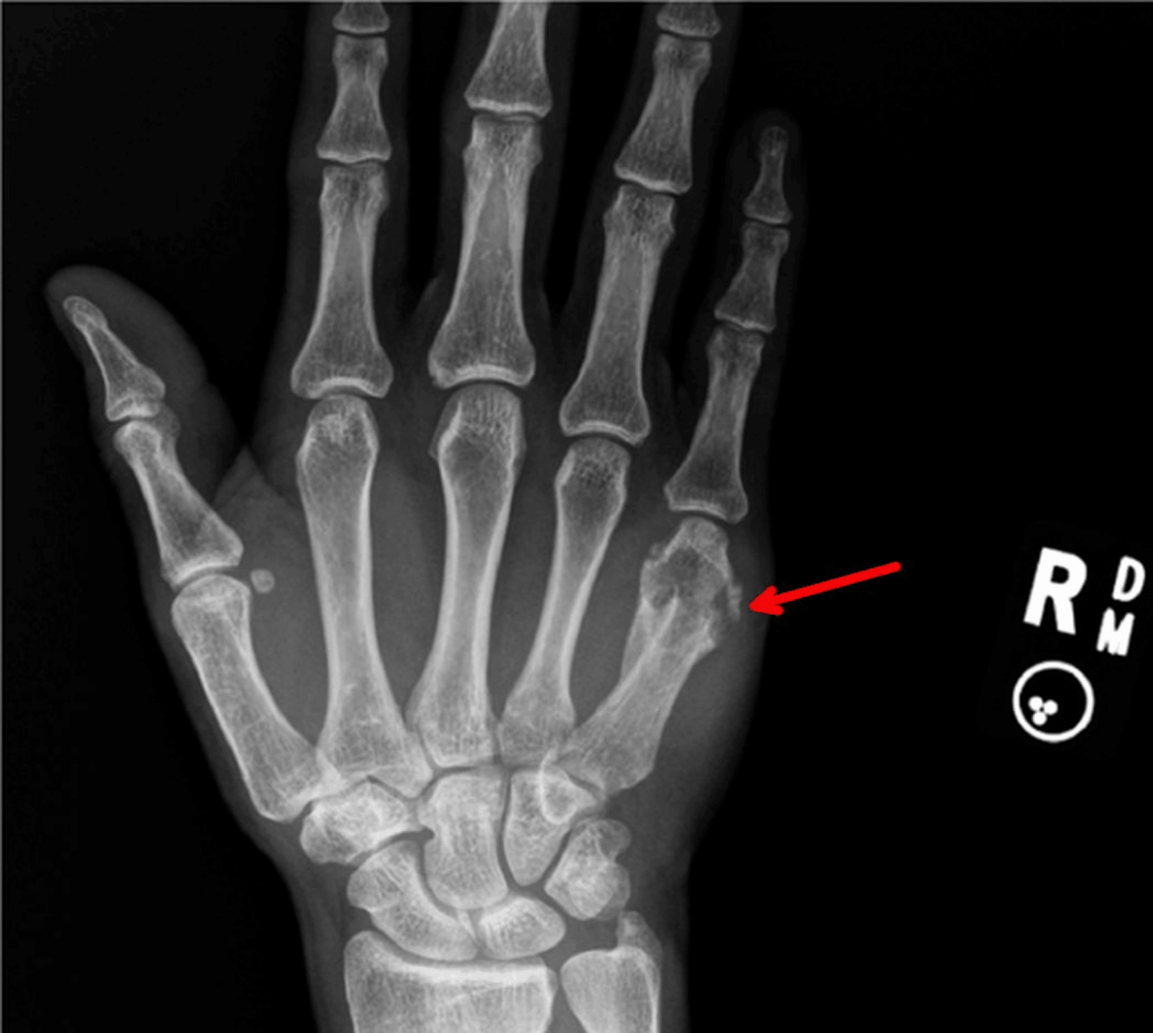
X-ray of the right hand shows lytic changes in the distal fifth metacarpal (arrow)
